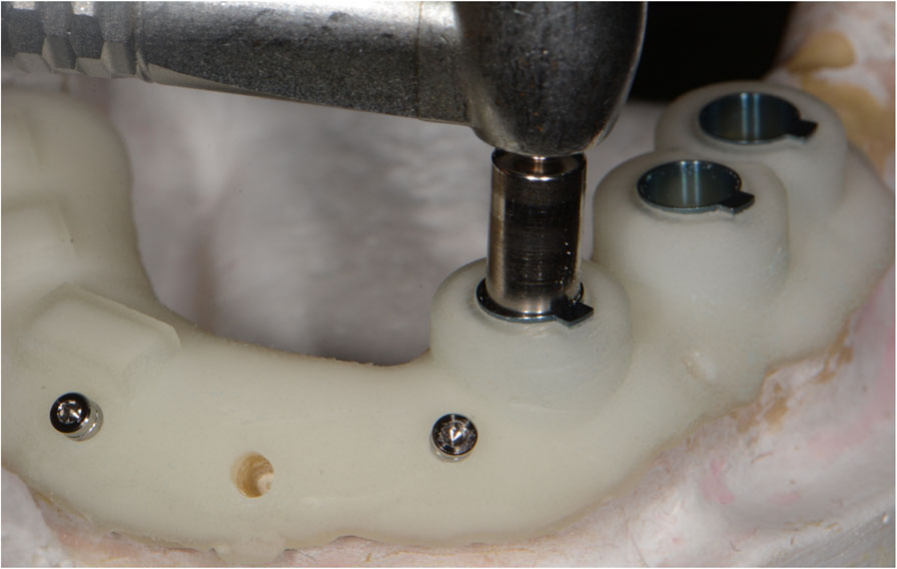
The surgical guide for implant placement. CBCT and CAD/CAM are used to produce a surgical guide for implant placement
